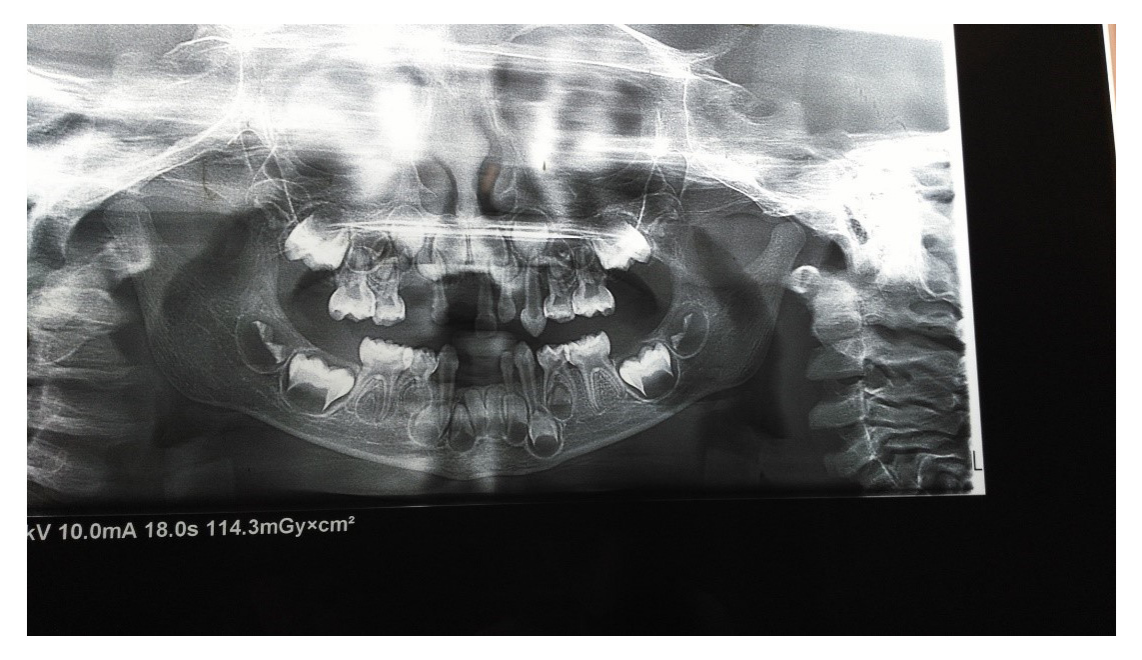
Panoramic radiograph showing severe destruction and loss of alveolar bone in both maxillary and mandibular arch, especially in the anterior region and anterior teeth appear as if floating in air without surrounding alveolar bone.
